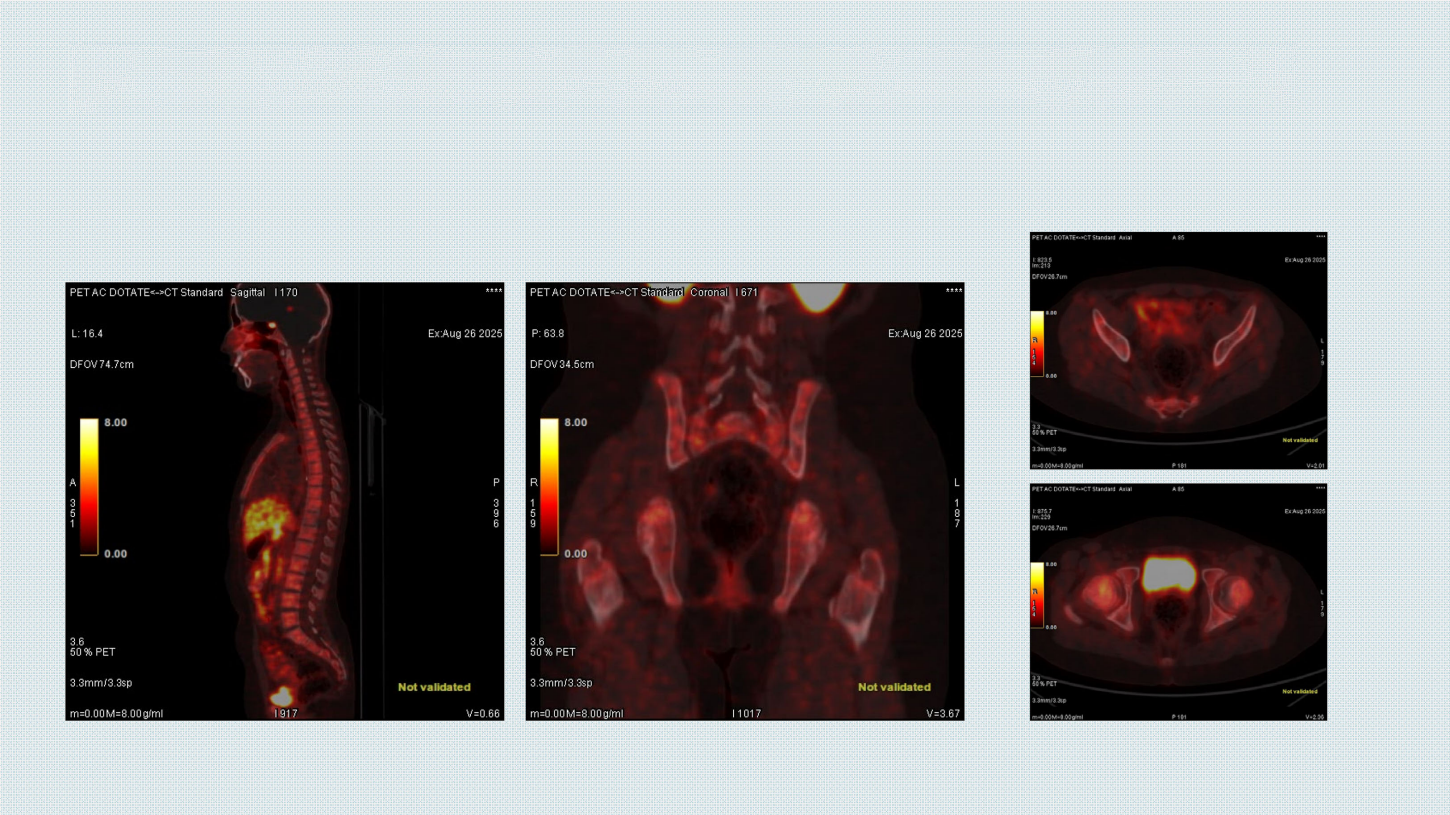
Improvement in bone involvement on DOTA-PET/CT image after combination treatment with lorlatinib and naxitamab.
